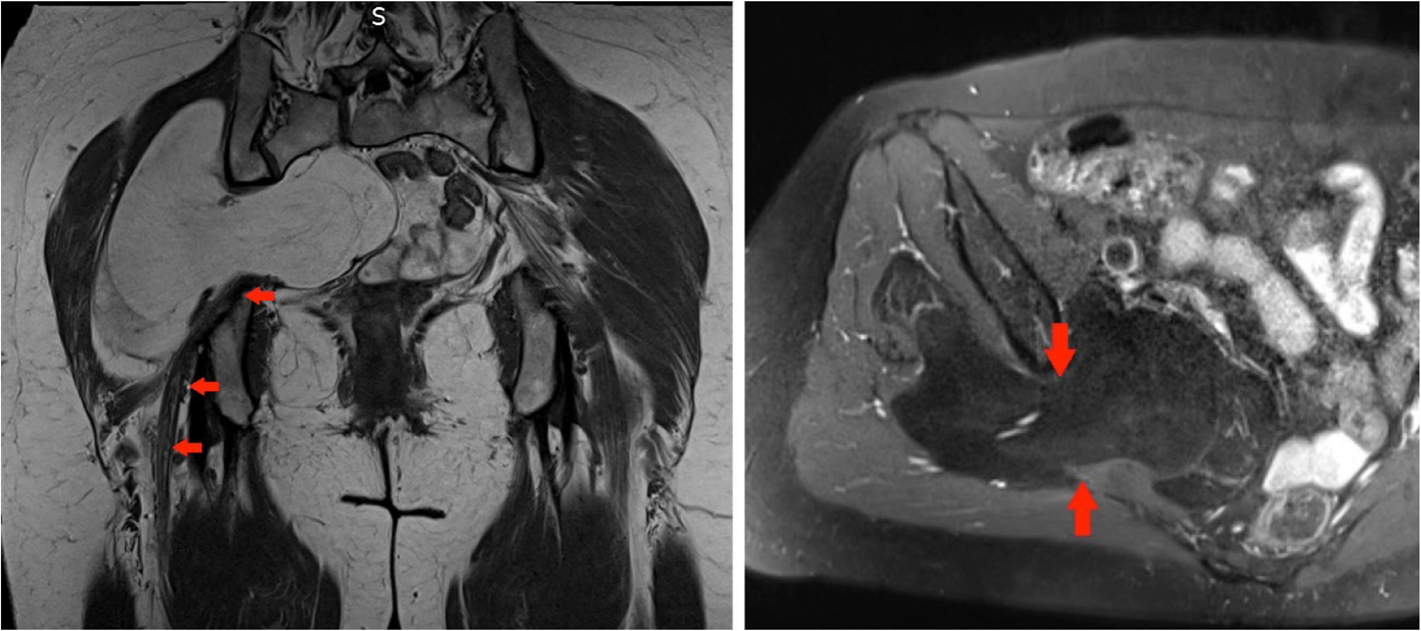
Left panel: Coronal T1-weighted MR image of the pelvis shows displacement and kinking of the right sciatic nerve at the level of the posterior acetabulum (red arrows). Right panel: Axial proton density fat sat weighted image of the pelvis confirms the lesion is predominantly fat containing as it supresses on fat saturated sequences. High signal septations which do not supress can also be visualised. Red arrows outline the greater sciatic notch
